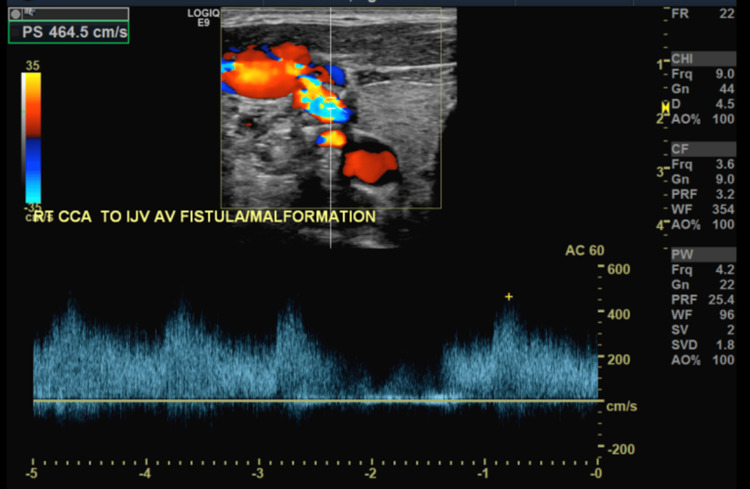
Carotid duplex ultrasound (color flow Doppler) R IJV: right internal jugular vein; R CCA: right common carotid artery; AV: arteriovenous
